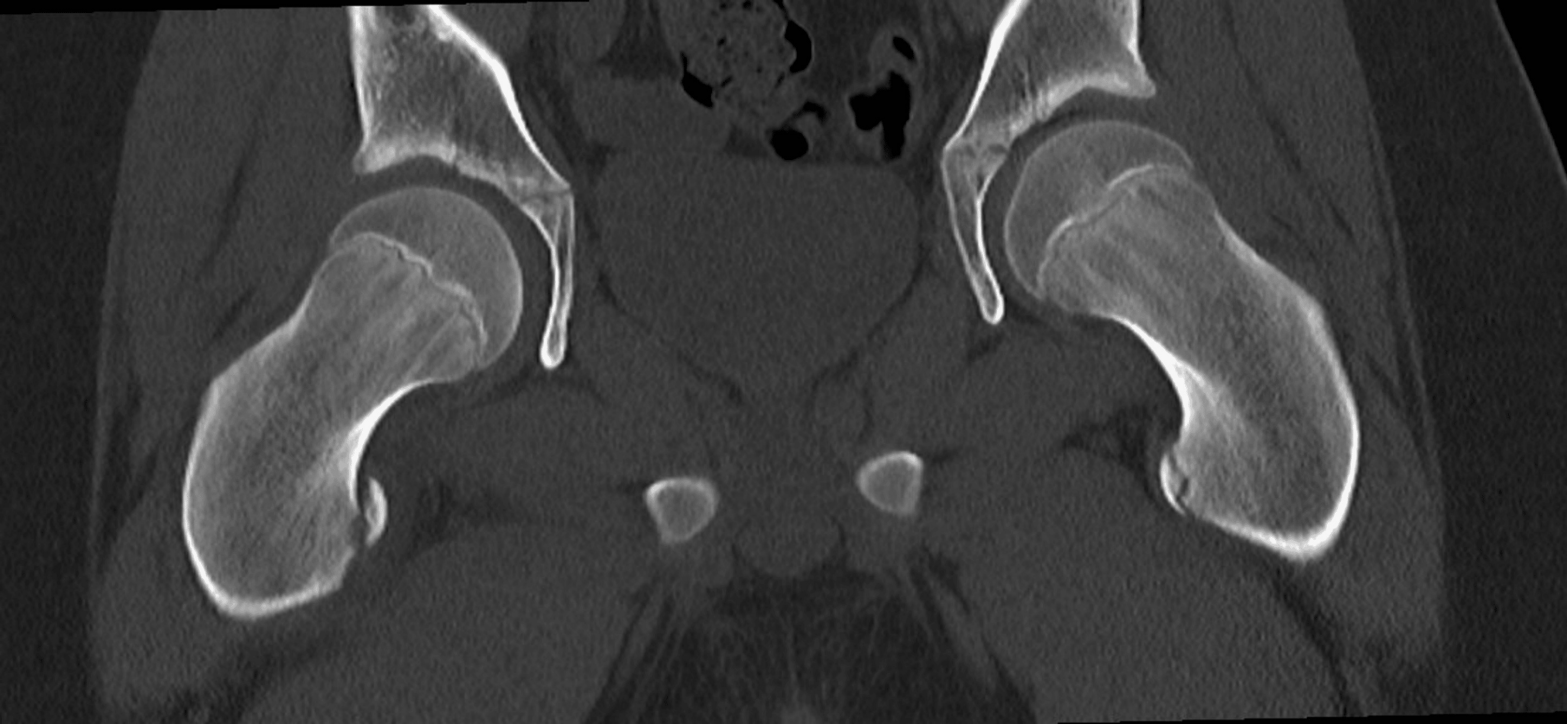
Pelvic CT after intraoperative exploration
